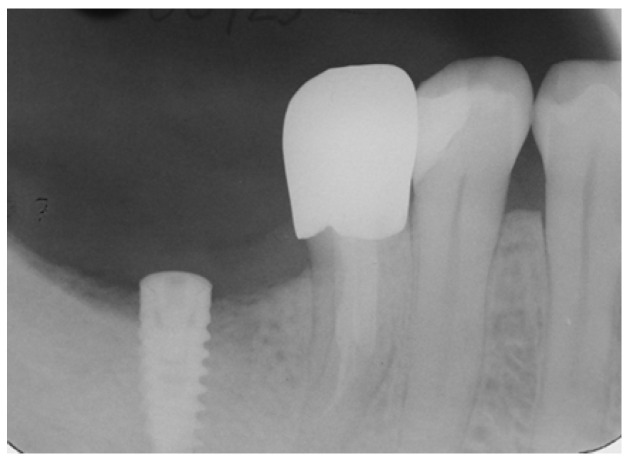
Periapical X-ray after implant placement showing osseointegration and the mesial root of #46 with normal bone tissues and absence of periapical pathology.
